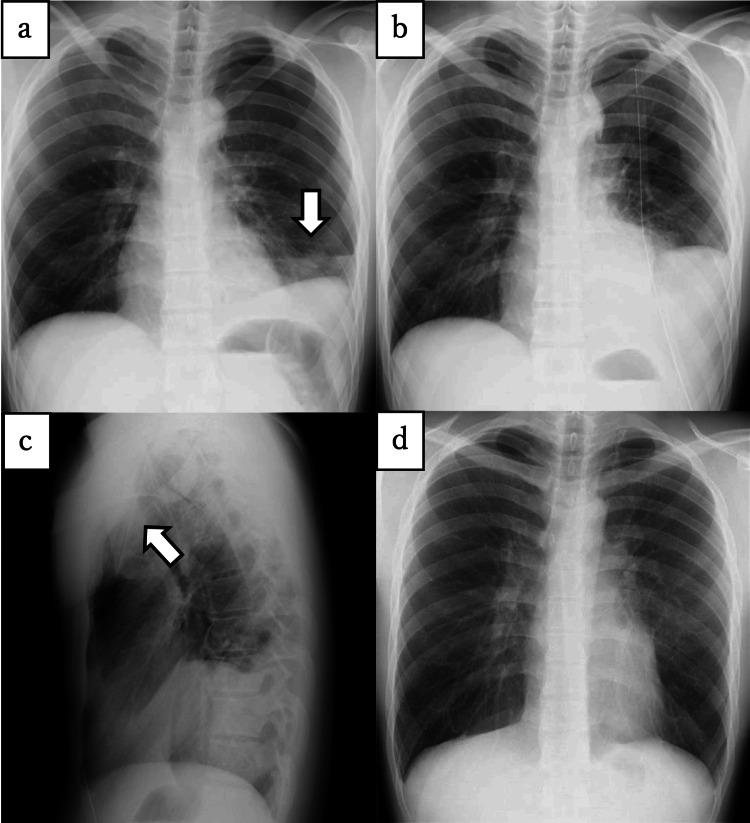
Representative case of delayed surgical intervention for spontaneous hemopneumothorax (SHP) (a) Initial chest X-ray showed moderate pneumothorax and small air-fluid level (arrow), but SHP was not suspected by primary care doctor. (b) Half a day later, after blood drainage presented. Follow-up X-ray revealed an increase of air-fluid level and the patient was diagnosed with SHP. (c) Chest tube was positioned ventrally (arrow); therefore, the hemorrhage drained belatedly. (d) Postoperative chest X-ray.
